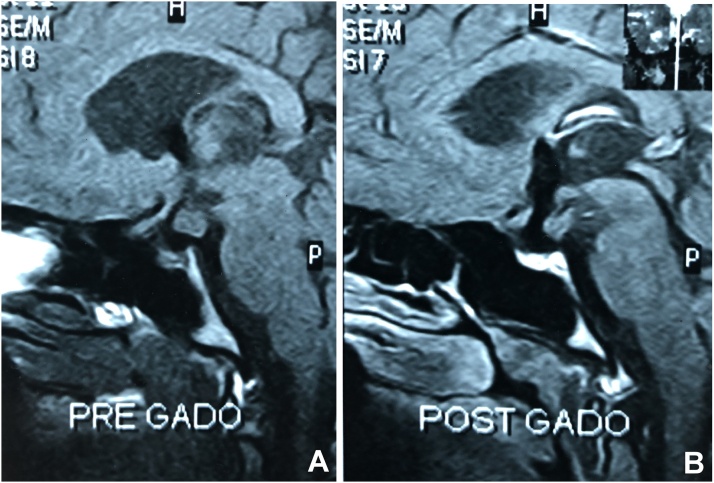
Preoperative MRI of the brain showing an evidence of suprasellar hypothalamic lesion suggestive of hamartoma.
